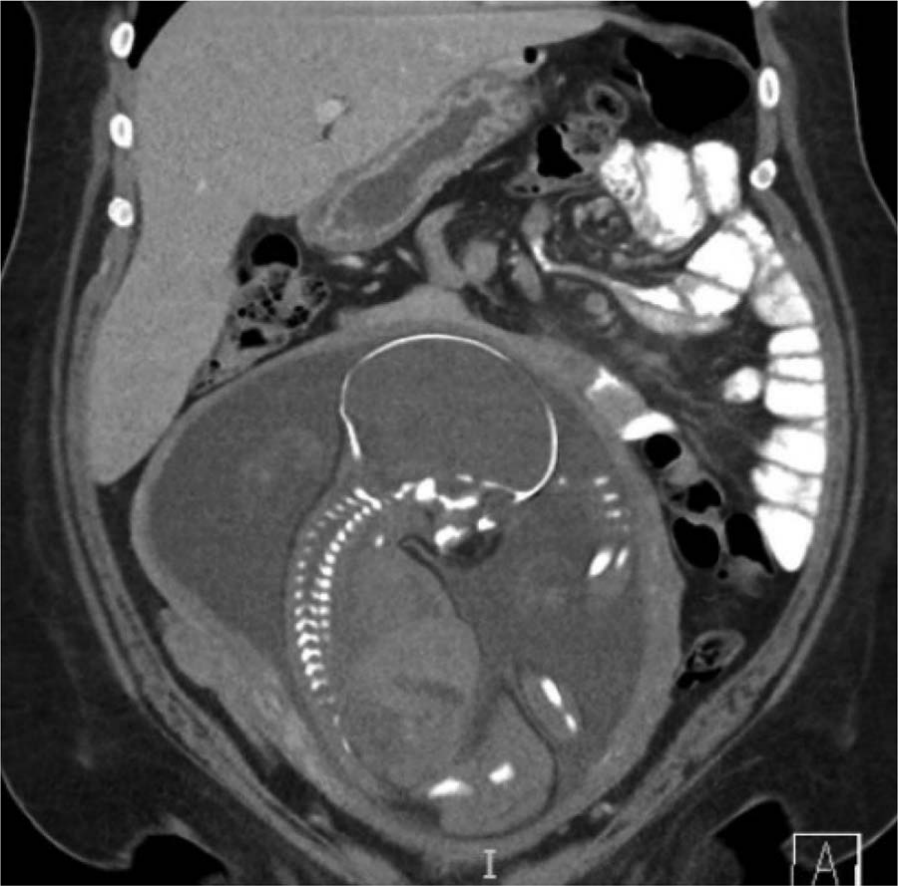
Coronal CT demonstrating left upper quadrant mesenteric swirling with cluster of small intestine entirely on the left abdomen.
